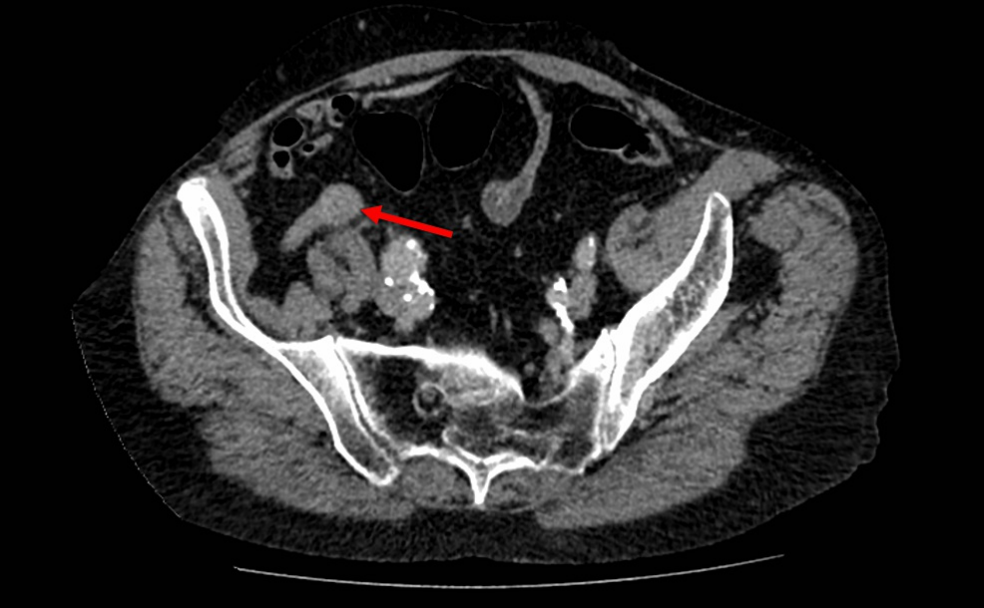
CT of the abdomen and pelvis - transverse section. A transverse cross-sectional CT image of this patient showing a fusiform appendiceal mass (red arrow). Other findings remain as described in Figure 1.
